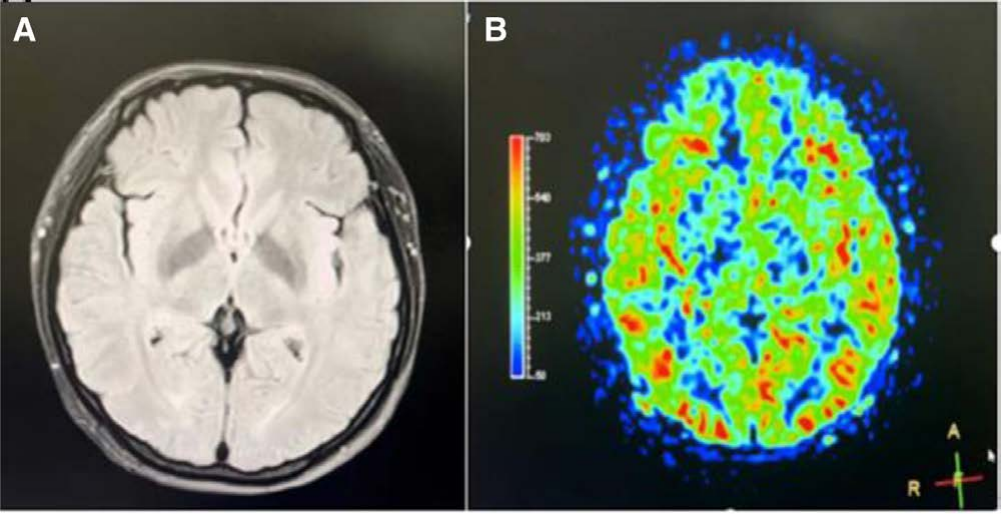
The cranial imaging findings of the patient following treatment and subsequent alleviation of clinical symptoms. (A) The abnormal signal intensity in the brain has resolved on MRI scan. (B) There are no significant abnormalities in cerebral blood perfusion on functional MRI. MRI = magnetic resonance imaging.
